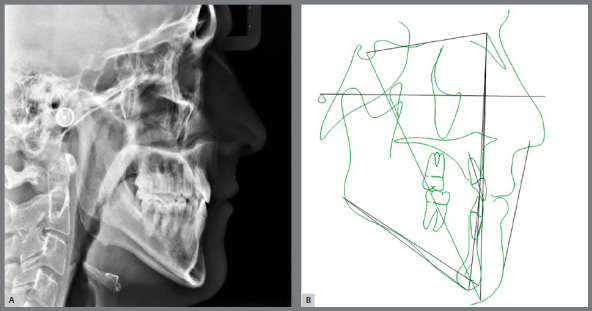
Cephalometric profile radiograph (A) and cephalometric tracing (B) 8 years after treatment.
